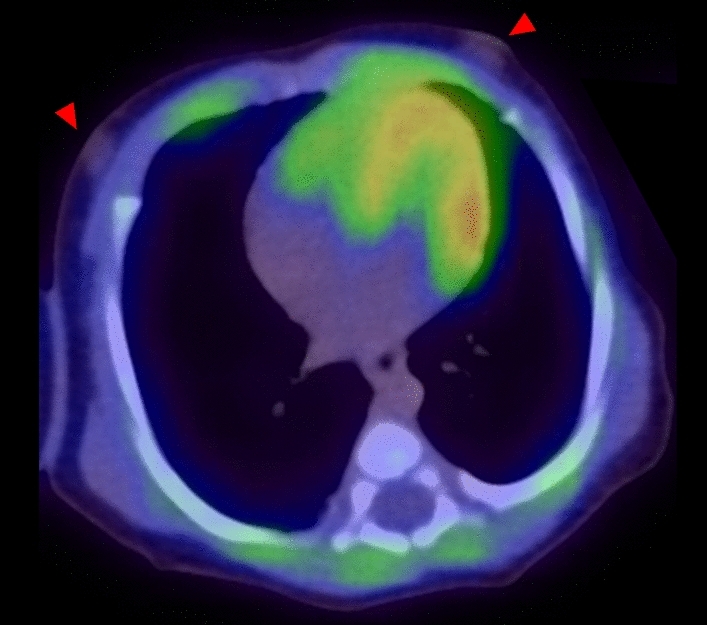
FDG PET/CT image of infants with 38 days after birth. Breast enlargement (red arrowhead) and FDG uptake in left ventricular wall
